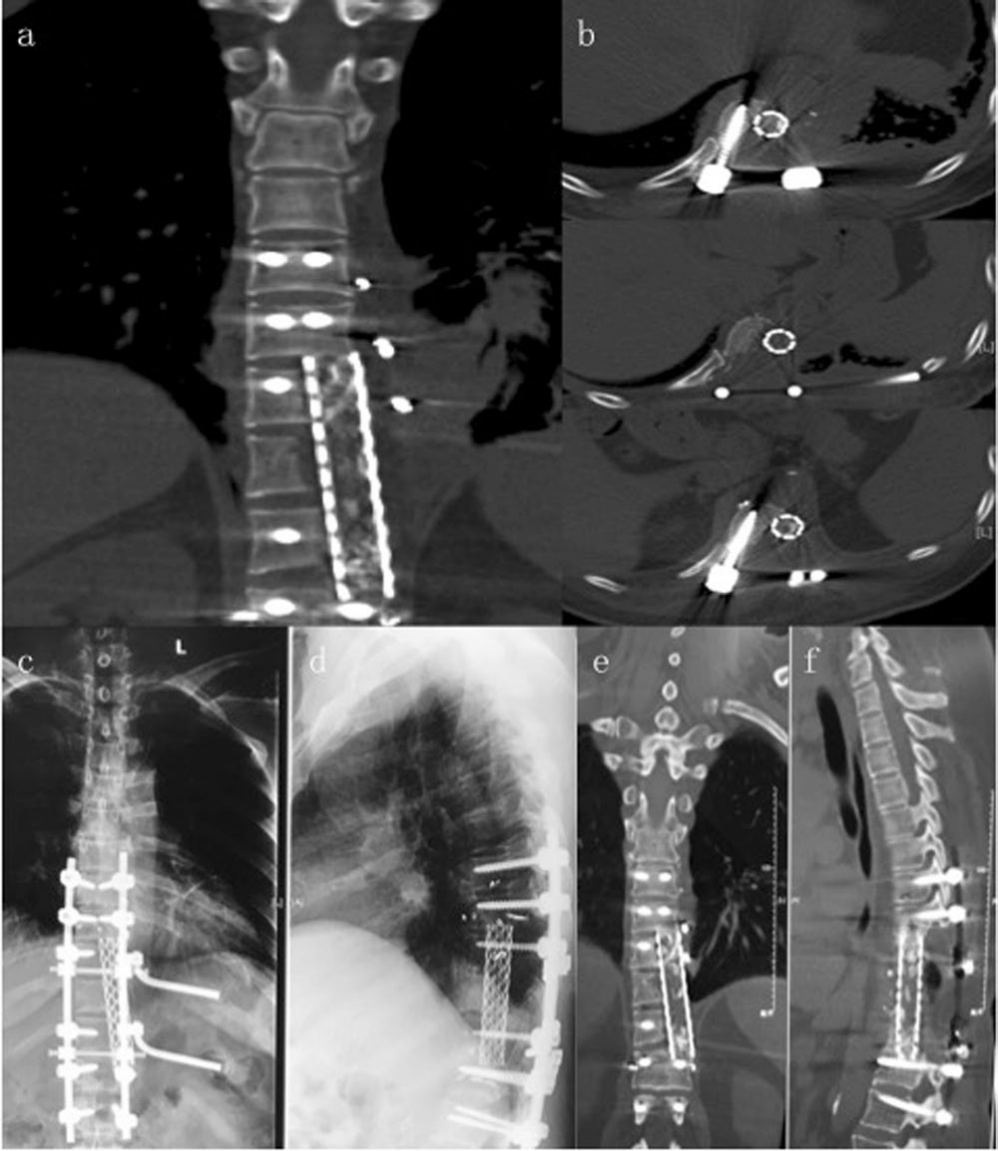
(a,b) Postoperative CT scans show structural reconstruction with instrumentation after sagittal resection. (c–f) Radiographs (c,d) and CT scans (e,f) taken at 2 years after surgery show no signs of hardware failure or tumor recurrence.
